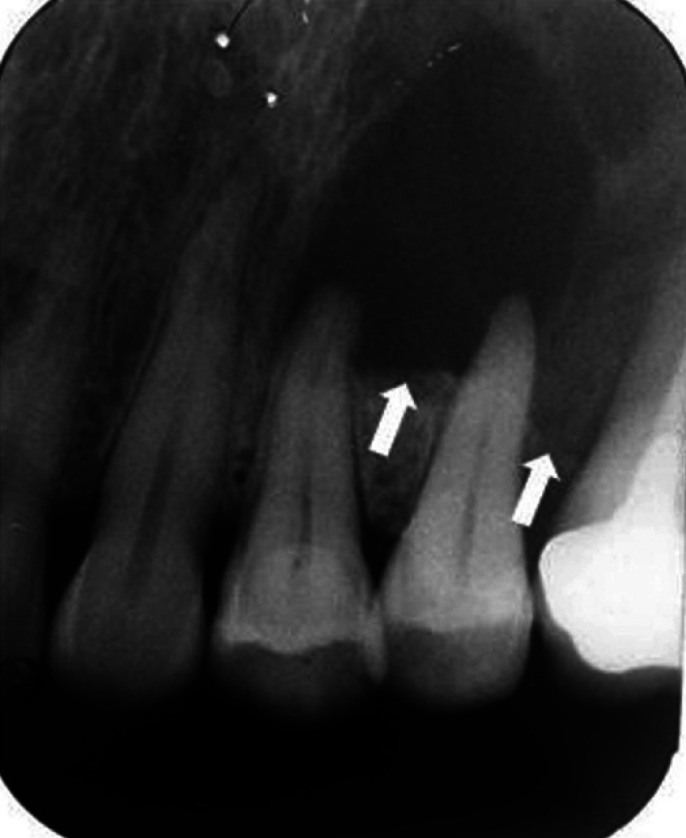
A periapical radiograph of a central mucoepidermoid carcinoma shows ill-defined radiolucent area at the left posterior maxillary region. Inferior border of the lesion is indicated by arrows. The superior and posterior borders of the lesion cannot be revealed on this periapical radiograph.
